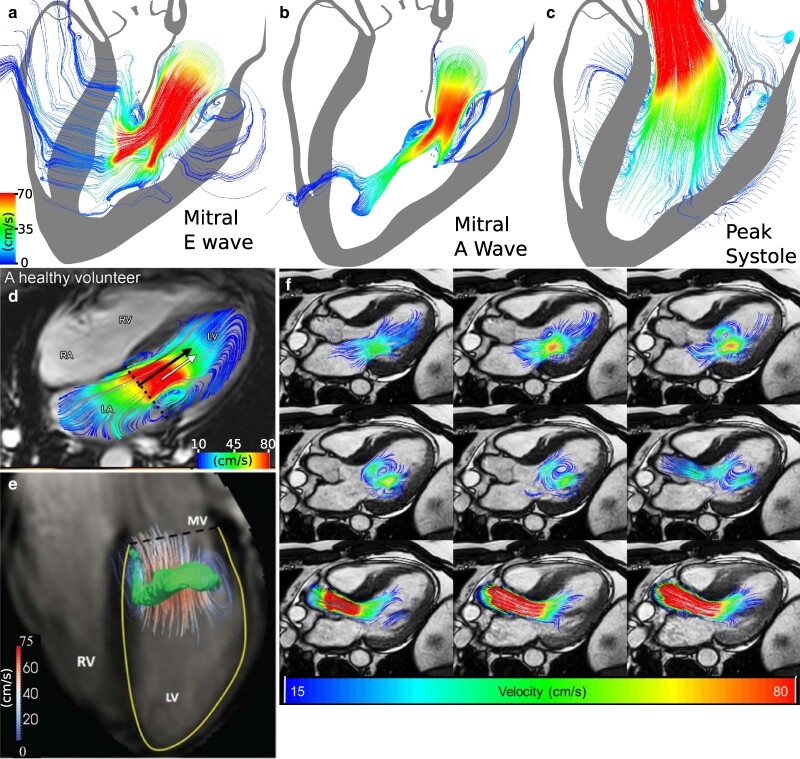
Comparison of instantaneous flow patterns generated by the model to in vivo data. Instantaneous streamlines are shown along two-dimensional slices that bisect the mitral valve and left ventricular outflow tract during peak E wave a), A wave b), and systole c). Notice that instantaneous streamlines passing through the heart wall are physical and reflect the wall motion. d) Streamlines generated from 4D flow MRI of a healthy subject during the E wave (adapted from Calkoen et al. (79)). e) Streamlines generated from 4D flow MRI of a healthy subject during the E wave with a superimposed vortex ring generated from $\lambda_{2}$-based methods (adapted from Elbaz et al. (61)). f) Streamlines generated from 4D flow MRI of a healthy subject from A wave onset (top left) to systolic ejection (bottom right) (adapted from Geest et al. (80)).
